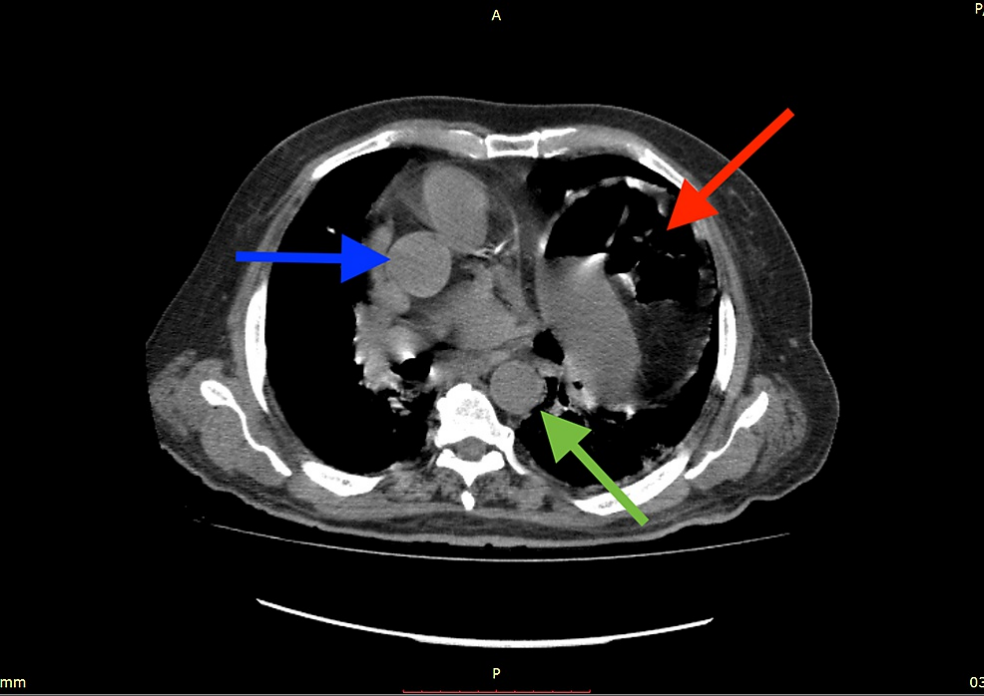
Transverse section chest HRCT showing the stomach and intestines with gas bubbles (red arrow) reaching up until the level of the arch of the aorta with ascending (blue arrow) and descending (green arrow) branches visible
